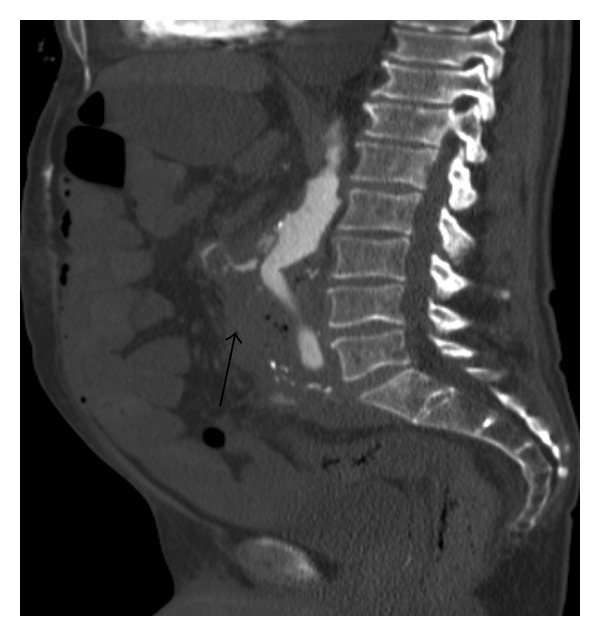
Sagittal contrast-enhanced CT scan of AEF.
